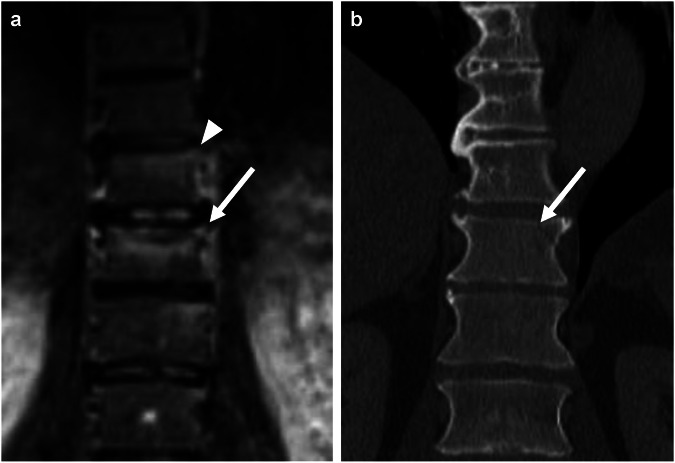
Coronary plane of a fracture of the 11th thoracic vertebra (white arrows). a Turbo Inversion Recovery Magnitude (TIRM) image (coronal MRI, 5 mm thick) showing a hyperintense line underneath the superior vertebral endplate and a hyperintensity underneath the superior vertebral endplates of the 10th and 12th thoracic vertebra, corresponding to bone bruise (white arrowhead). b CT of the same patient only depicted the fracture of the 11th thoracic vertebra
